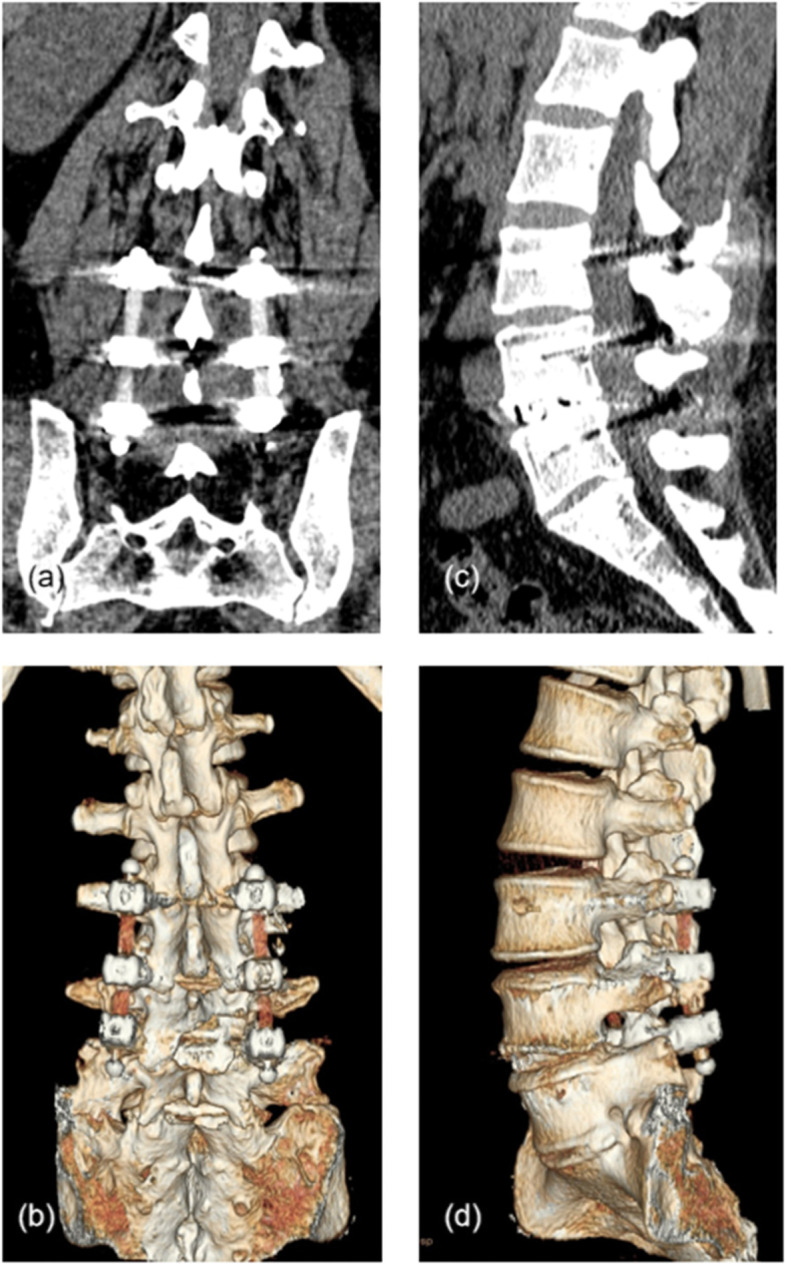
Typical case. a, b Coronal lumbar CT scan and 3D reconstruction taken at the 2-year follow-up, showing the contours of the bilateral PEEK rods. c, d Sagittal plane image and 3D reconstruction showing intervertebral fusion was achieved at the L4/5 segment
